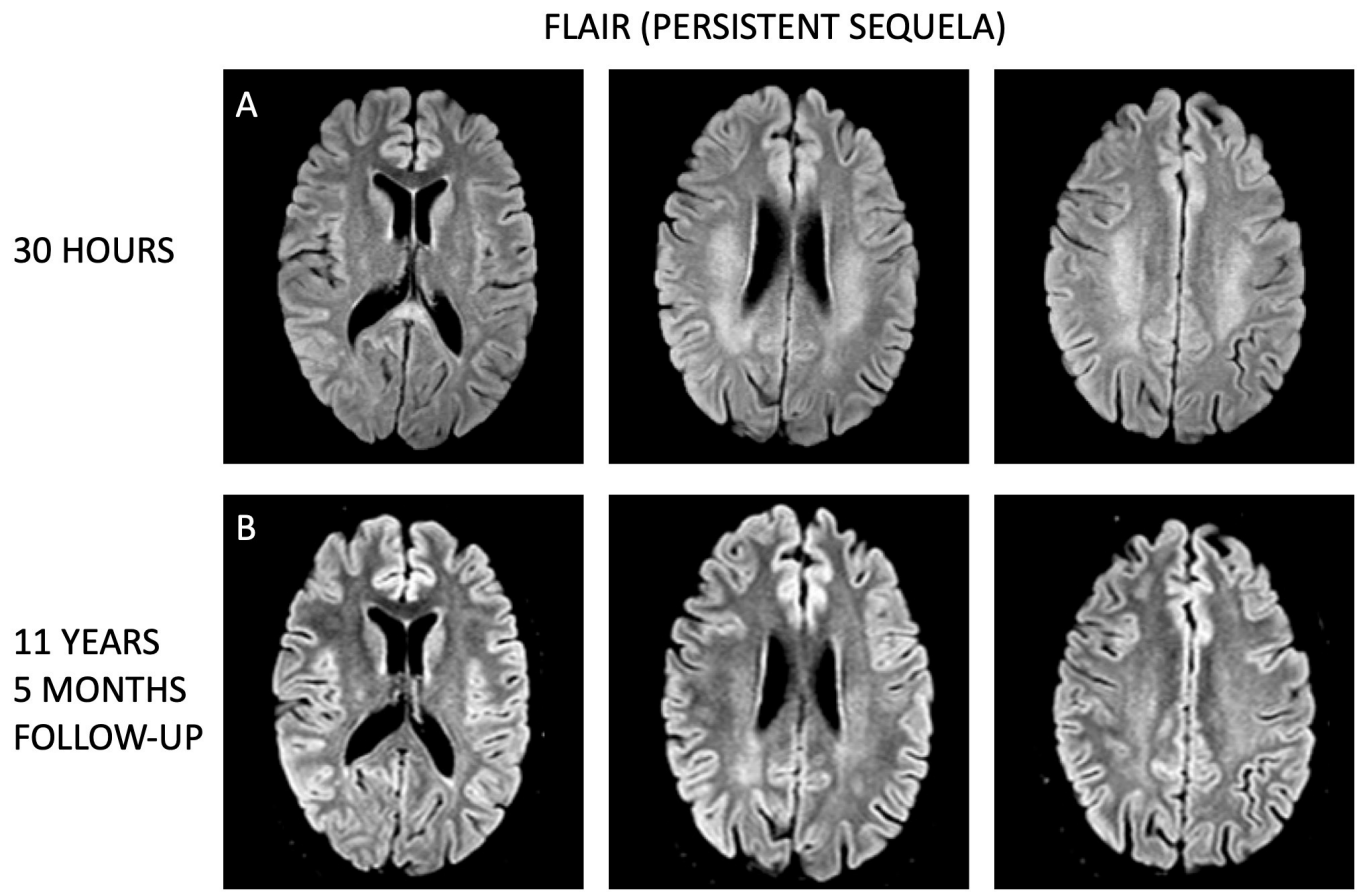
Longitudinal comparison of white matter lesions on fluid attenuated inversion recovery (FLAIR) MRI sequences. (A) Display axial sections from the initial MRI taken 1 day after the transient neurological symptoms, while (B) show corresponding axial sections from the follow-up MRI conducted 11 years and 5 months later. Despite the time gap, the white matter lesions remain visible more than a decade after, although less prominent.
